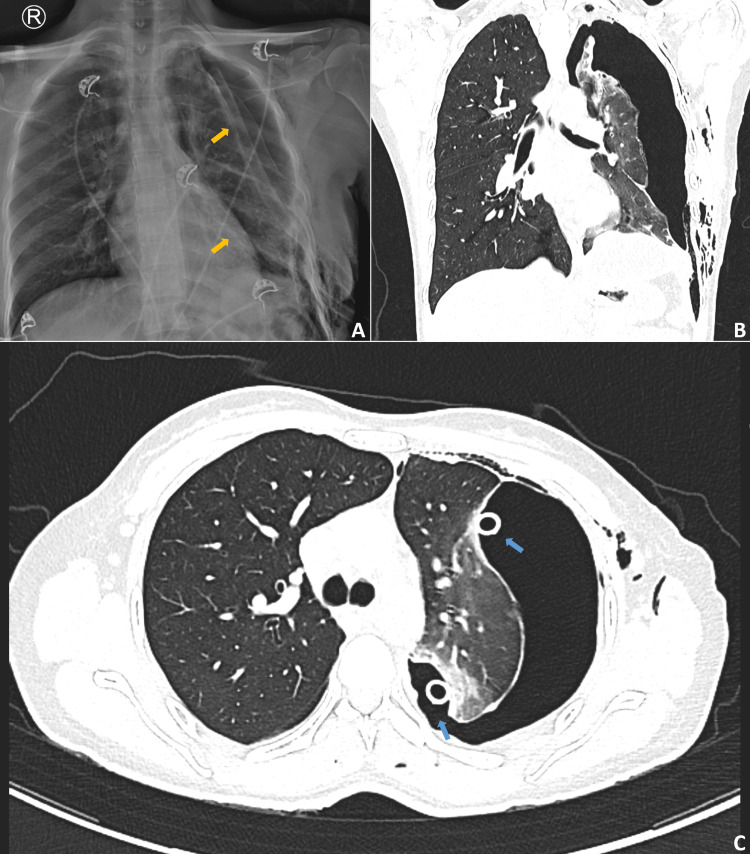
Postoperative imaging findings (A) Chest radiograph obtained six hours after VATS-assisted deroofing of left pleural hydatid cysts, showing a left-sided pneumothorax with two chest tubes in situ (yellow arrows). (B, C) Contrast-enhanced CT chest (postoperative day one) demonstrating a large left-sided pneumothorax with compression of the left lung parenchyma and two chest tubes in the left pleural cavity (blue arrows).
